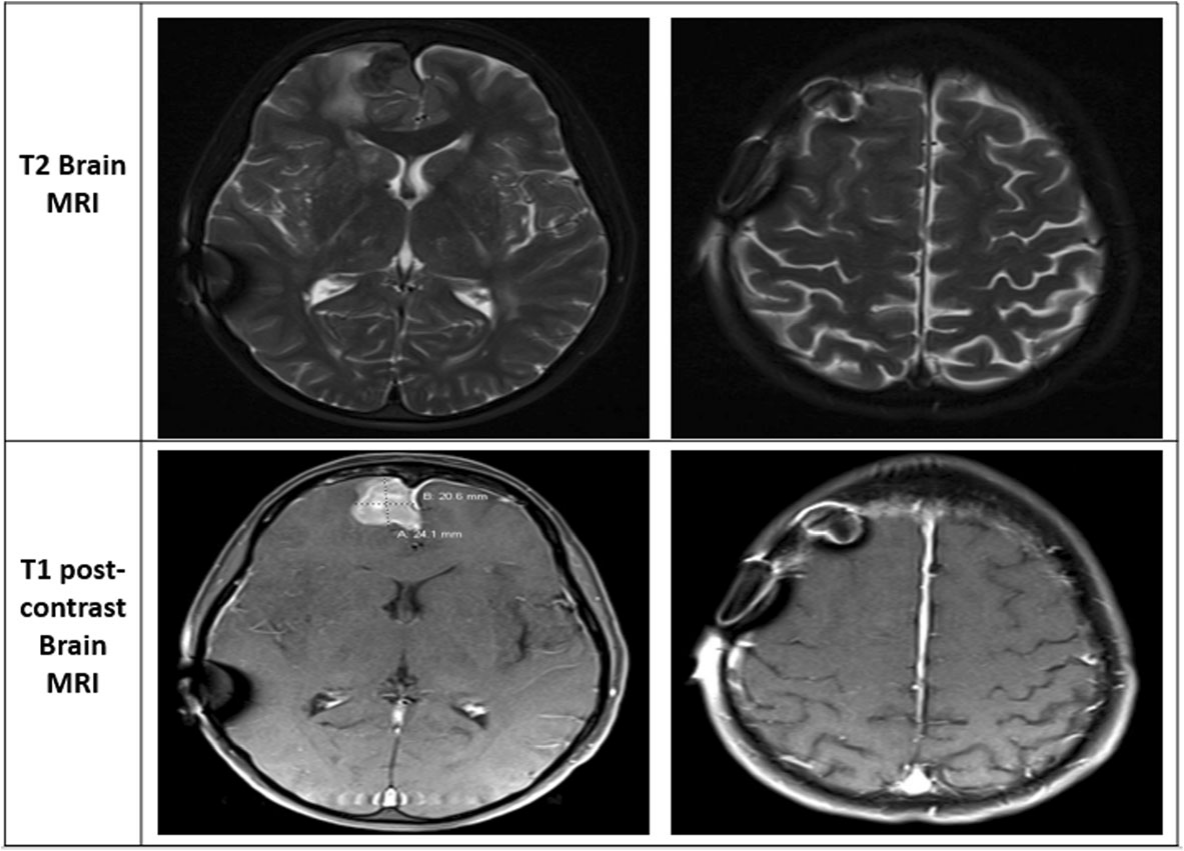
Magnetic resonance images (MRI) showing multiple enhancing intracranial lesions and extradural enhancing lesions consistent with radiation-induced meningiomas
